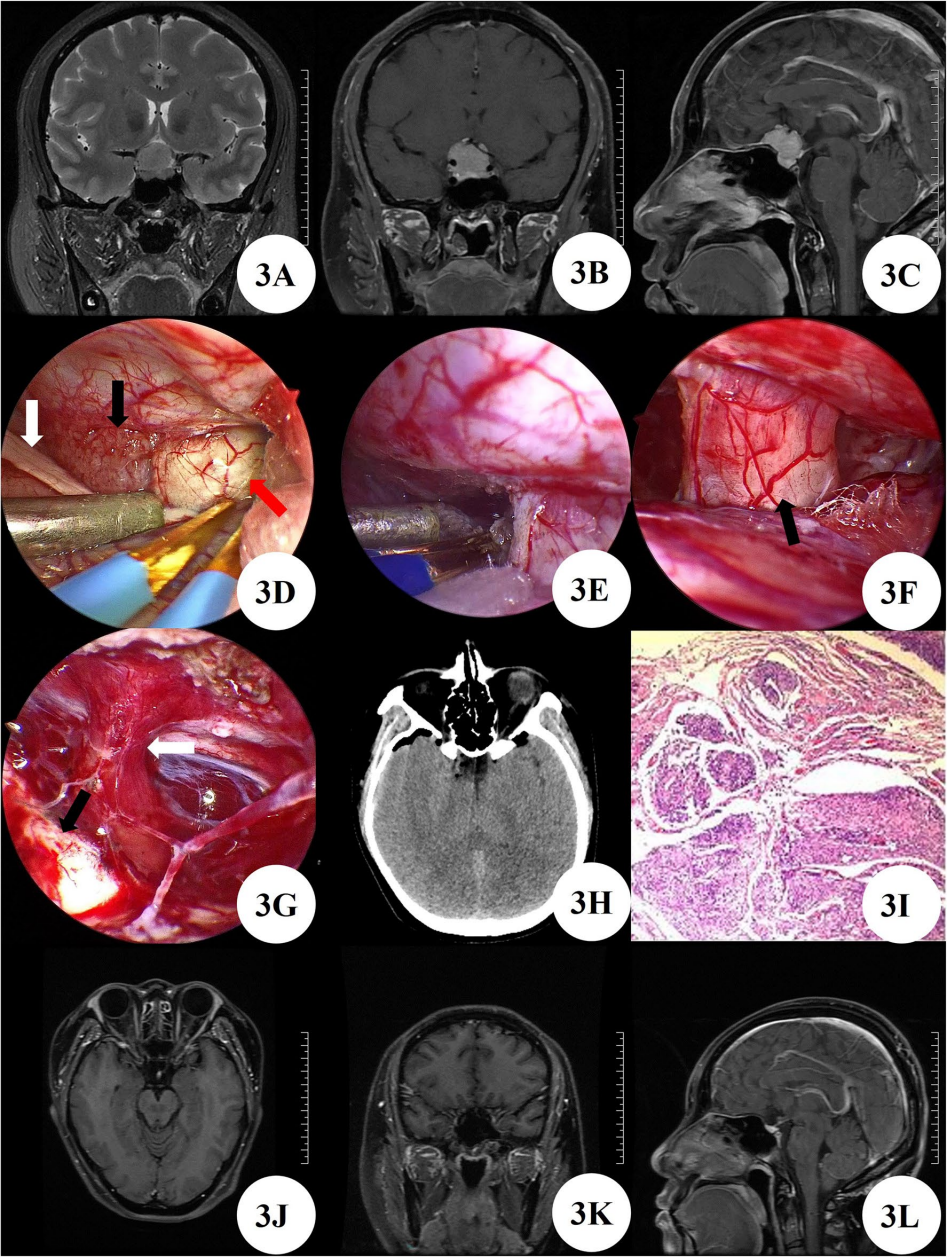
(A–C) Preoperative MRI shows mass lesions in the sellar region and suprasellar region. (D) Tumor (Black arrow), olfactory nerve (White arrow), and optic nerve visible (Red arrow) by neuroendoscopy. (E) Detachment of tumor base. (F) The right optic nerve is well preserved (Black arrow). (G) The pituitary stalk (White arrow) and left optic nerve (Black arrow) are well preserved. (H) Postoperative CT shows tumor resection with no bleeding in the surgical area. (I) Pathological examination diagnosed meningioma. (J–L) Follow up MRI at 8 months post-surgery showed no recurrence of the tumor.
